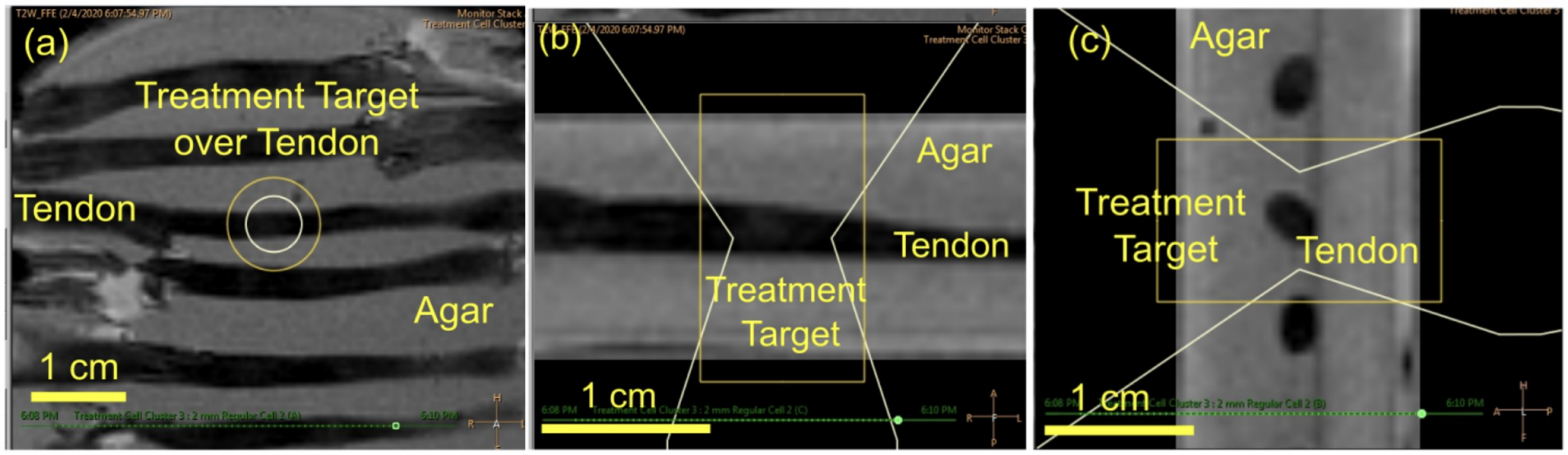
T2-w MRI scan of tendons in agar mold and MRgFUS treatment target focused on treatment target for (a) coronal, (b) transverse, and (c) sagittal planes.
